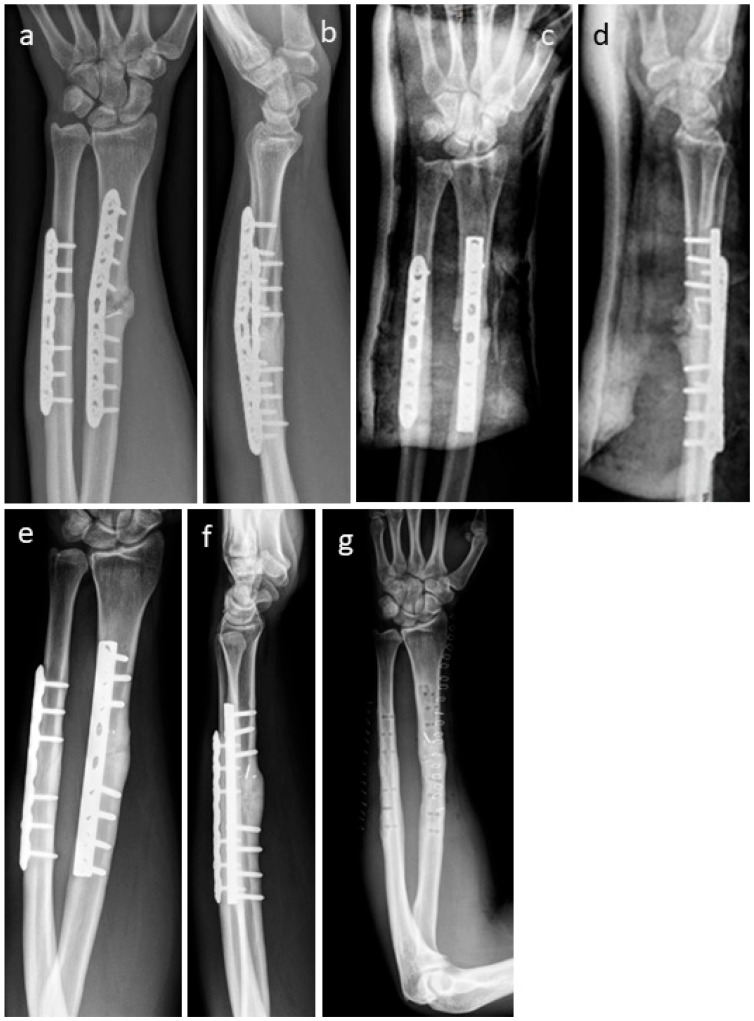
The non-union of a radius in a patient is shown; (a,b): pre-revision X-rays. As the former radius plate was bent, the radius was shortened in relation to the ulna. During the revision operation, the plate on the radius was replaced and the radius was restored to its original length. The resulting bone defect was filled with an allogeneic cortical block and DBM putty. In addition, the bone defect was stabilized and bridged with two Shark Screws^®^. (c,d): Post-surgery X-rays; union was obtained after 12 weeks. (e,f): Before metal hardware removal, one year after revision surgery. (g): After metal removal.
